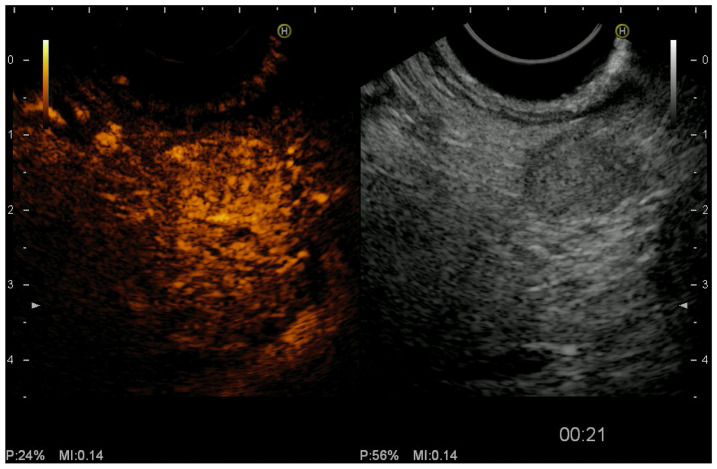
Endoscopic ultrasound (EUS) revealed a 10 mm hypoechoic lesion of the pancreas (right panel). Contrast-enhanced EUS showed typical homogeneous hyperenhancement of the lesion during the arterial phase in comparison to the surrounding pancreatic parenchyma (left panel) after intravenous injection of contrast agent (Sonovue™, Bracco Imaging, Milan, Italy). P, power; MI, mechanical index.
